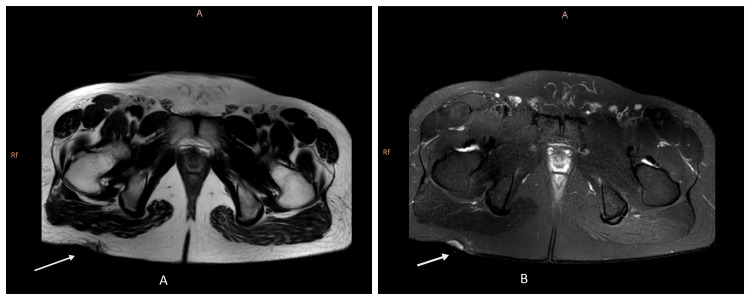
MRI gluteal region axial view A and B indicate a heterogenous signal intensity lesion seen in the subcutaneous plane in the right gluteal region, measuring approximately 21 x 8 x 21 mm. Underlying muscles are normal. No extension was seen into the pelvic cavity or underlying bones. Underlying neurovascular bundles and sciatic nerves are normal. No abnormal flow void was seen within the lesion. Multiple small inguinal lymph nodes are seen. Suggestive of mitotic pathology.
